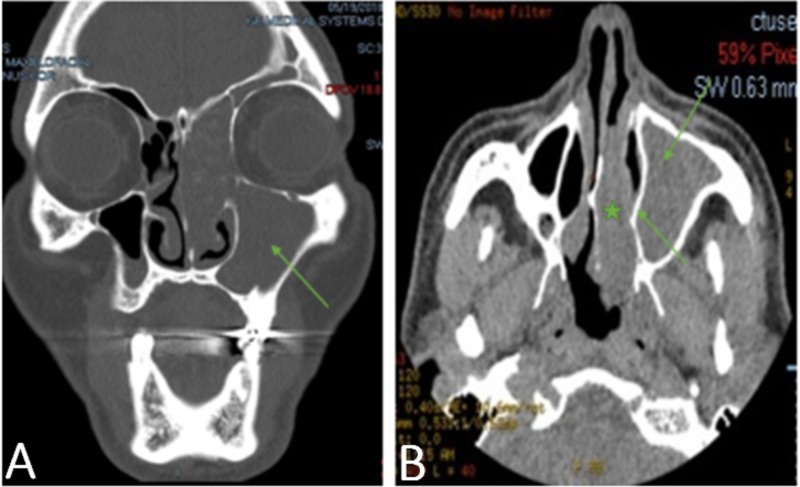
Non-contrast maxillofacial computed tomography (CT) scan Coronal (Figure A) and axial (Figure B) non-contrast maxillofacial CT scan demonstrates near-complete opacification of the left ethmoid and maxillary sinus, suggesting malignancy involving maxillary sinus and the left nasal cavity. Figure B shows septum deviation secondary to mass effect.
